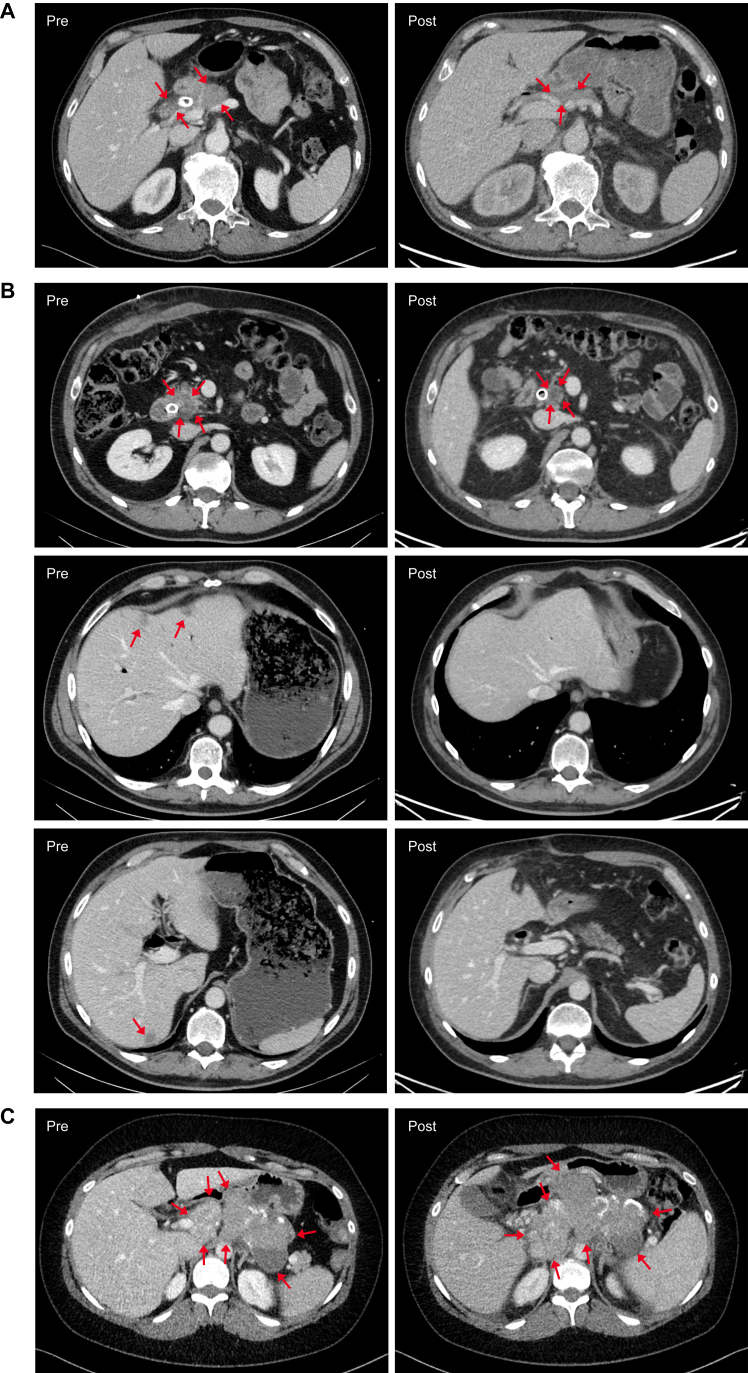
CT images for three patients who experienced benefit from treatment. (A) Patient PC-9 with locally advanced pancreatic cancer was treated with LOAd703 5 × 10^11^ VP combined with gemcitabine and nab-paclitaxel. The images show pancreatic tumor size at baseline (Pre) and 7 months after study inclusion with partial response according to RECIST1.1 (Post) with a decrease of 57%. (B) Patient PC-10 with liver-metastasized pancreatic cancer treated with LOAd703 5 × 10^11^ VP combined with gemcitabine and nab-paclitaxel. The top row of images shows pancreatic tumor size at baseline (Pre) and 6 months after study inclusion with partial response according to RECIST1.1 (Post) with a total decrease of 71%. The second and third row show images of liver metastases from the same time points. (C) Patient OC-5 with metastasized ovarian cancer treated with LOAd703 1 × 10^11^ VP, initially combined with gemcitabine. Gemcitabine was discontinued during study participation due to side-effects. The images show lymph node metastases at baseline (Pre) and stable disease 9 months later (Post) according to RECIST1.1. The lesions followed according to RECIST 1.1 had then increased in size by 14%. CT, computed tomography.
